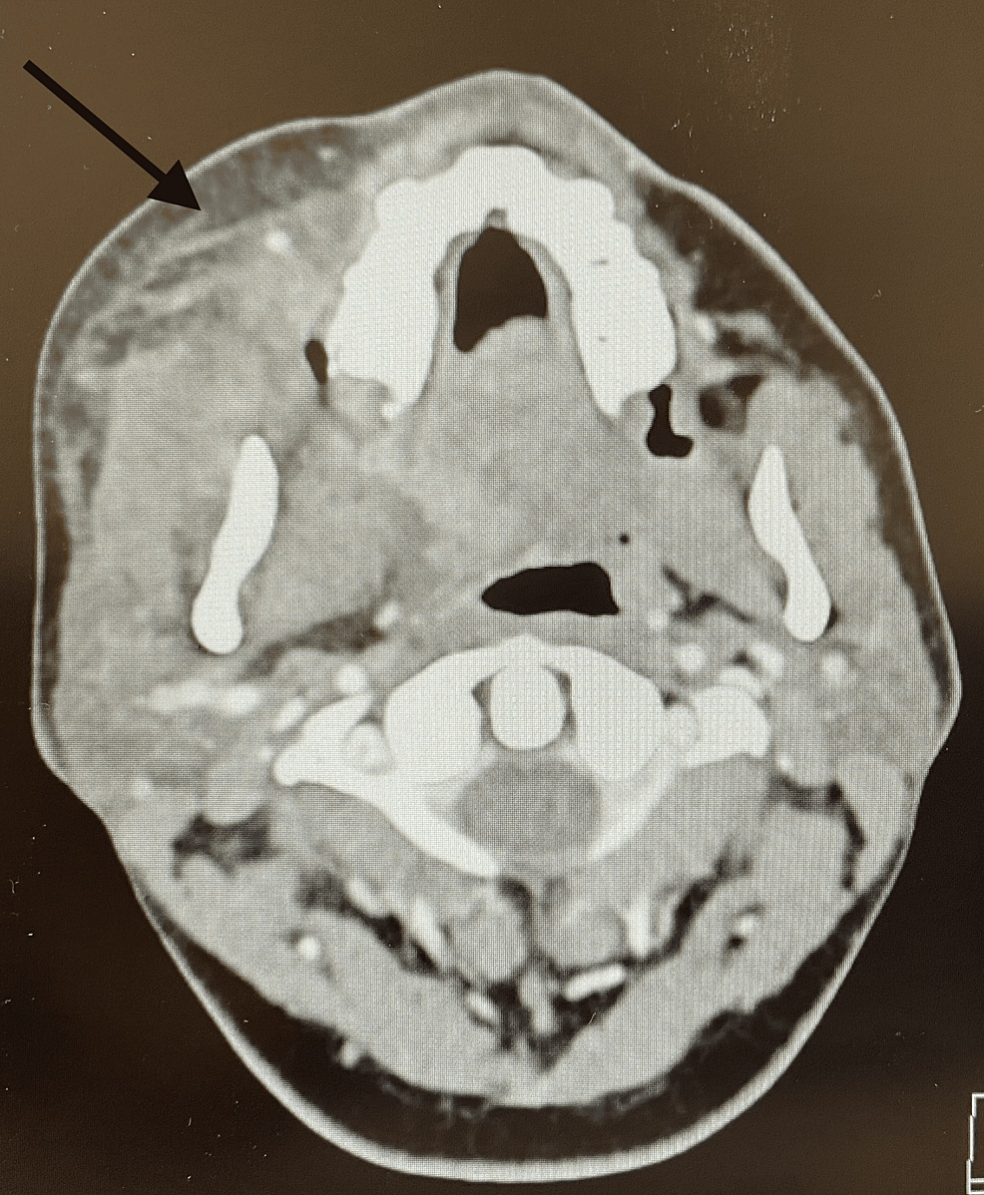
CT demonstrating severe edema of the pterygoid and masseter muscles with fat stranding.
